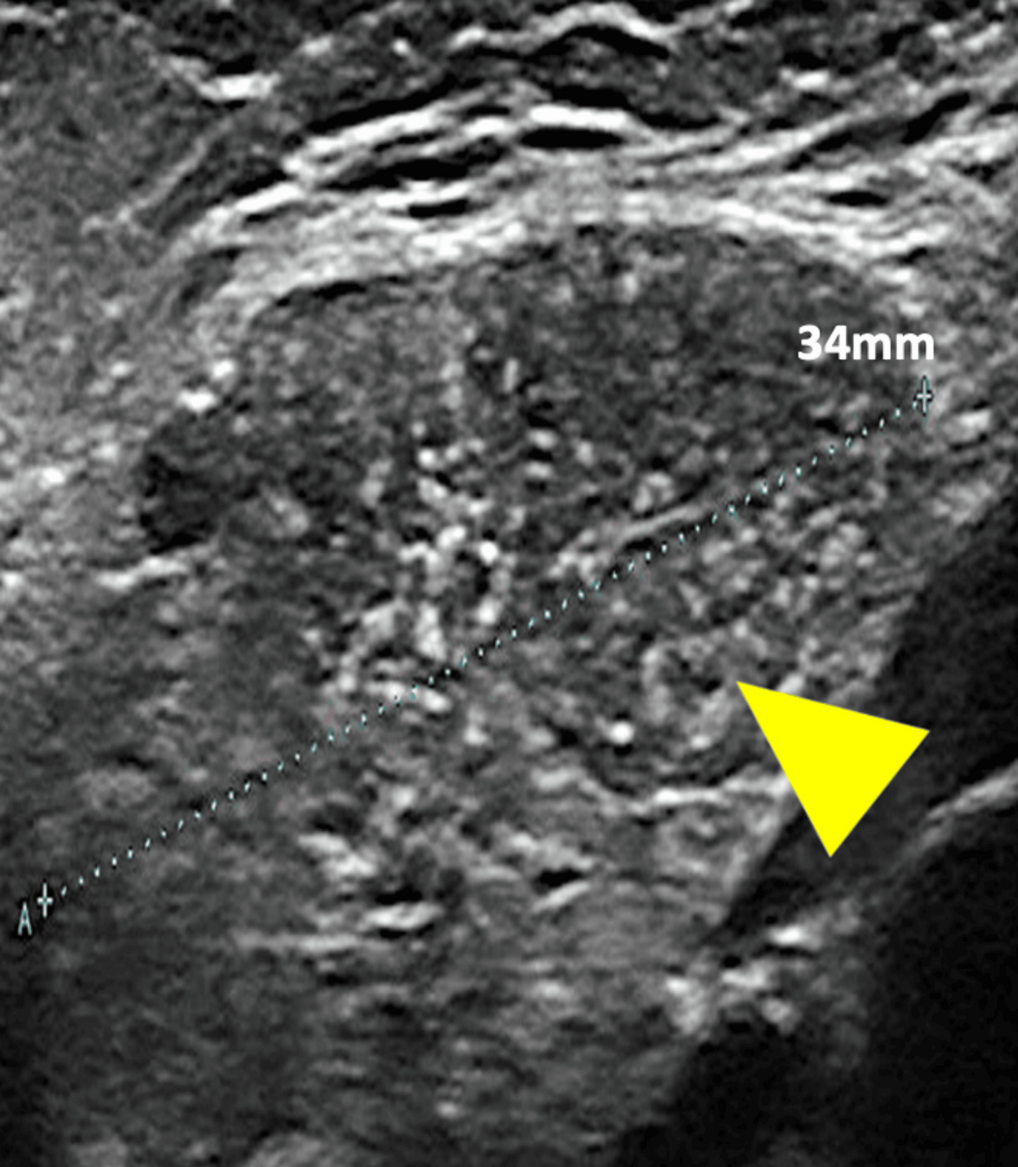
Ultrasound image of the cervical mass Ultrasound image (high-frequency linear probe, 11 MHz) of the cervical mass showing a well-defined hypoechoic mass measuring 34 mm in its longest diameter with a fine mosaic-like internal pattern of multiple branching echogenic linear structures and foci ("starry-sky" appearance) (yellow arrowhead).
